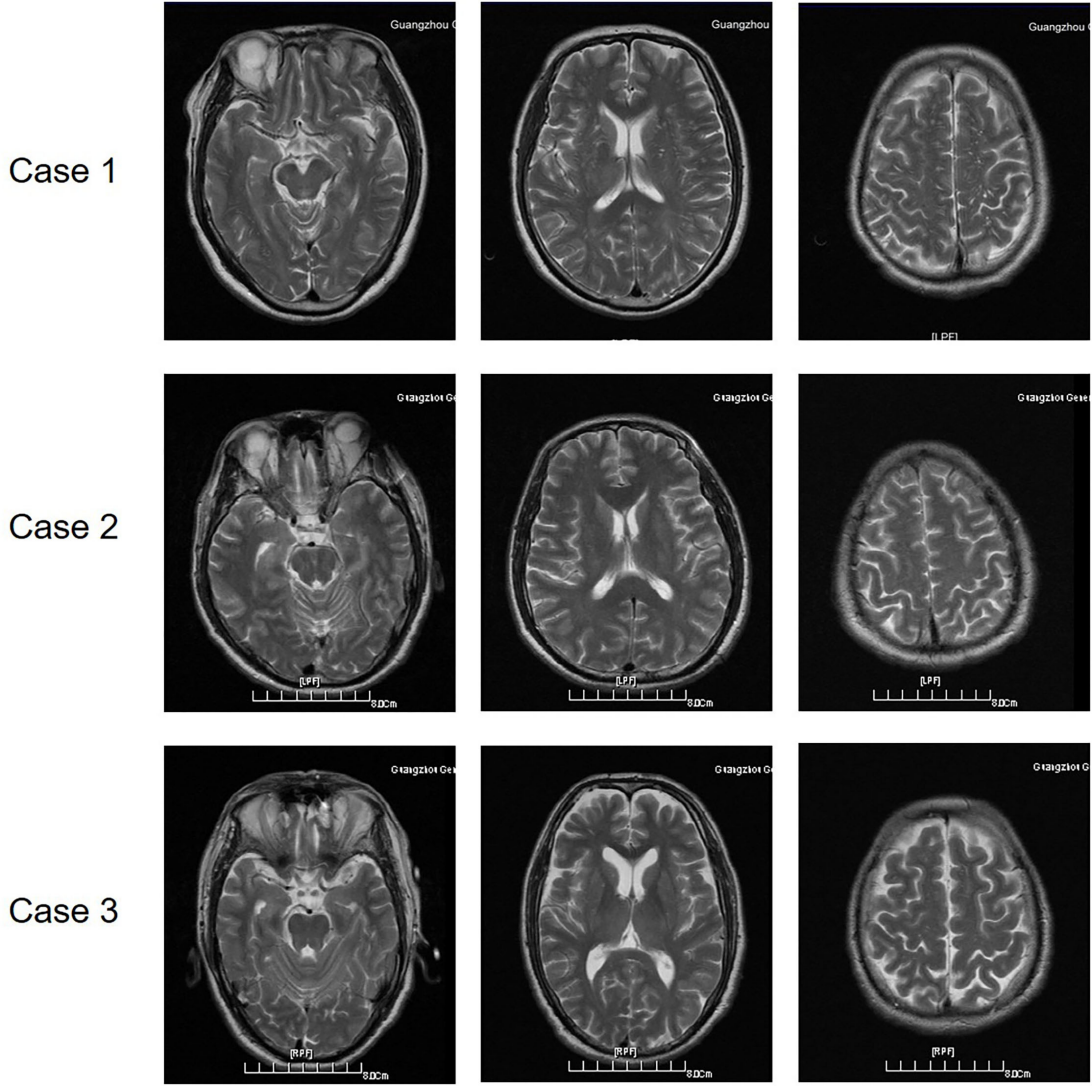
The brain magnetic resonance imaging (MRI) of the three patients with GBS induced by HS.
